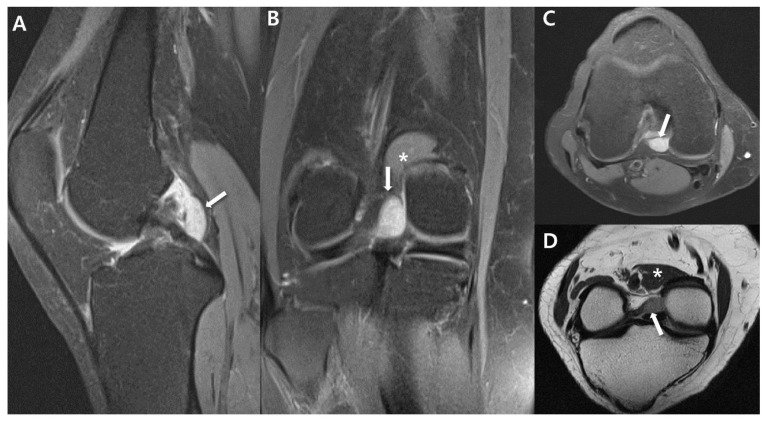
Intra-articular synovial sarcoma of 44-year-old-women (monophasic type): (A) Sagittal proton-weighted, fat-suppressed image shows about 2.0 × 1.2 × 3.0 cm sized circumscribed oval nodule (arrow) around posterior femoral recess of left knee. (B,C) Coronal and axial proton-weighted, fat-suppressed images show homogenous high signal intensity nodule (arrow) compared to adjacent muscle (*). (D) Axial oblique T2-weighted image shows a nodule (arrow) with relatively high signal intensity compared to muscle (*) but not bright high signal intensity such as ganglion cyst.
